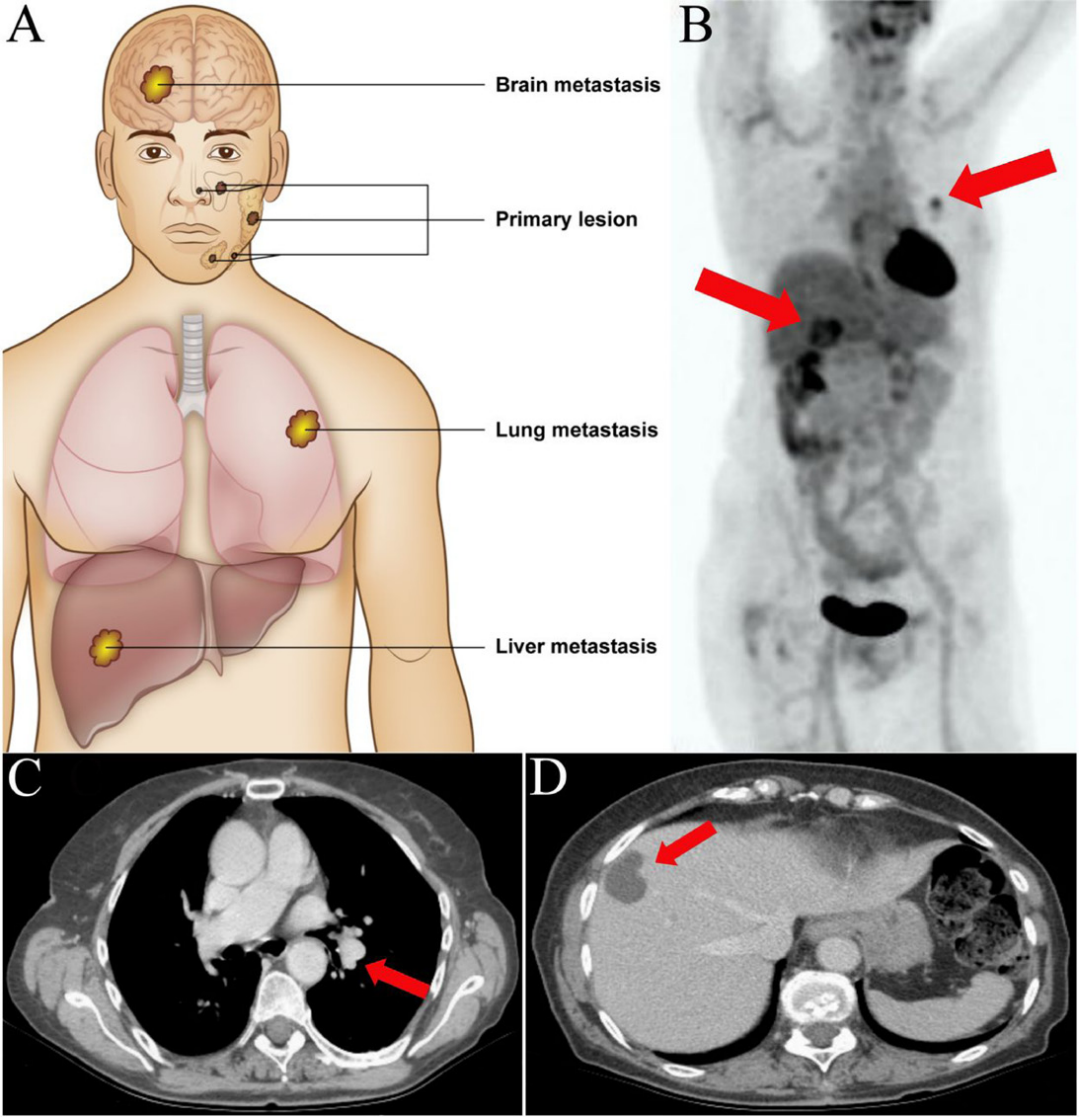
Anatomical localization of primary salivary gland adenoid cystic carcinomas and distant metastases (A) Primary adenoid cystic carcinomas were located to the major and minor salivary glands of the head and neck. Distant metastases were located to the brain (*n* = 1), lungs (*n* = 8), and liver (*n* = 3). (B) Positron emission tomography – computerized tomography (PET-CT) scan of case 6 with metachronous metastases to the hilar region of the left lung and liver (red arrows). (C) CT-scan of the same patient showing the pulmonary and (D) hepatic metastases.
